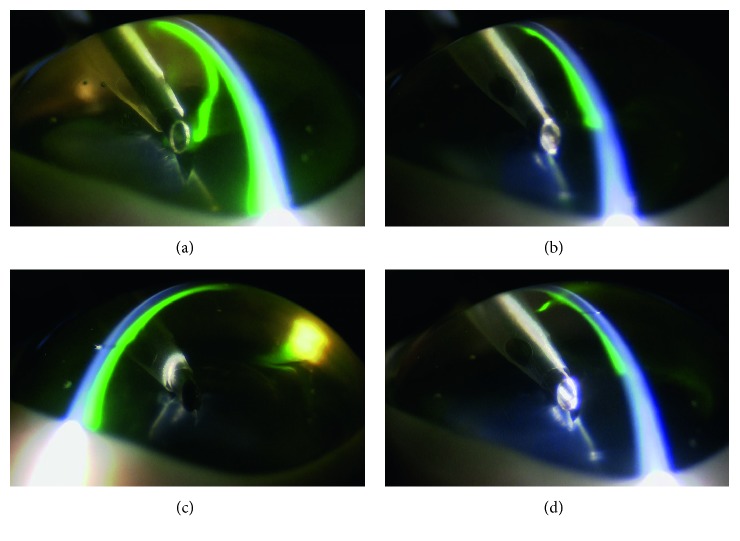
Soft-shell technique: (a) 3 seconds: after the application of ultrasound, the cohesive types of ophthalmic viscosurgical devices (OVDs) were immediately aspirated. (b) 25 seconds: the dispersive types of OVDs were retained on the surface of the corneal endothelium. (c) 30 seconds: cross-sectional view of the tip. (d) 90 seconds: dispersive types of OVDs formed a layer with a certain thickness on the surface of the corneal endothelium.
